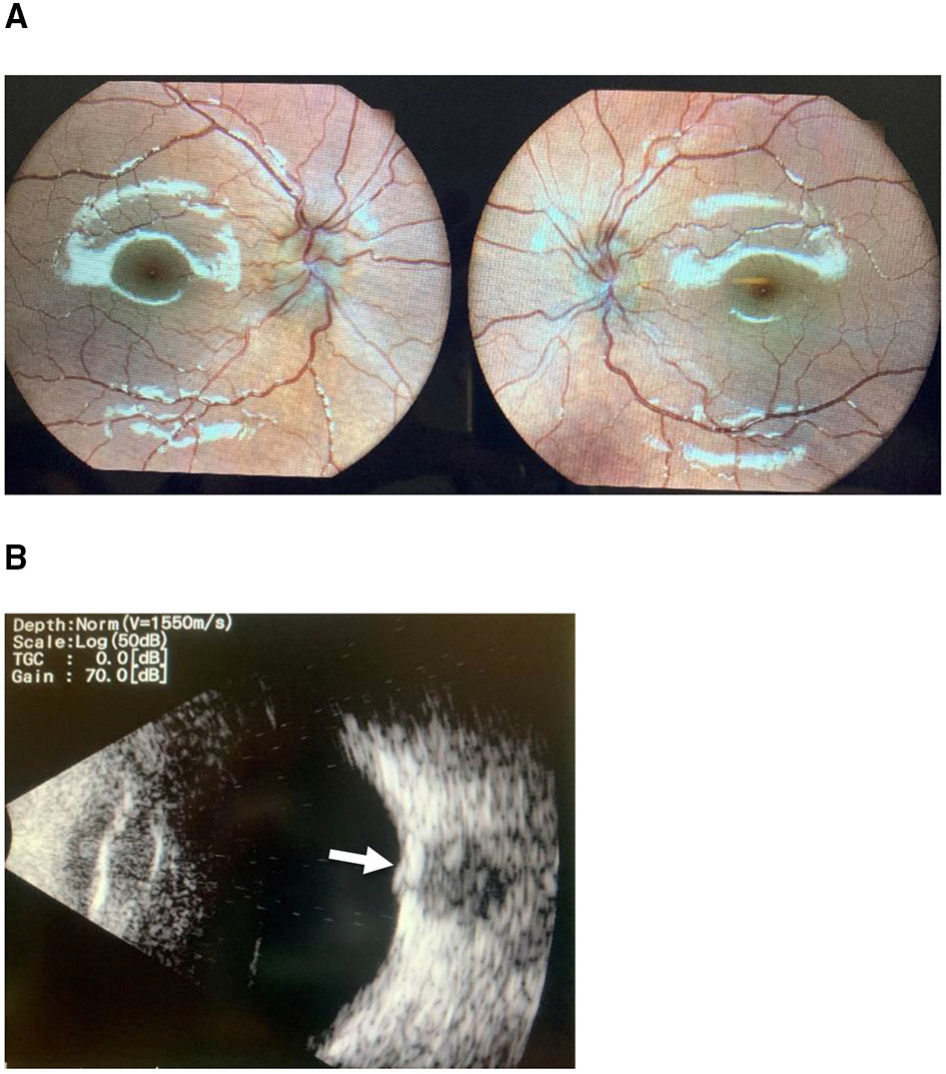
(A) Optic disc photography demonstrates papilledema. (B) B-scan ultrasonography confirming the presence of ahyper-reflective optic disc druse (arrow) in the same patient.
